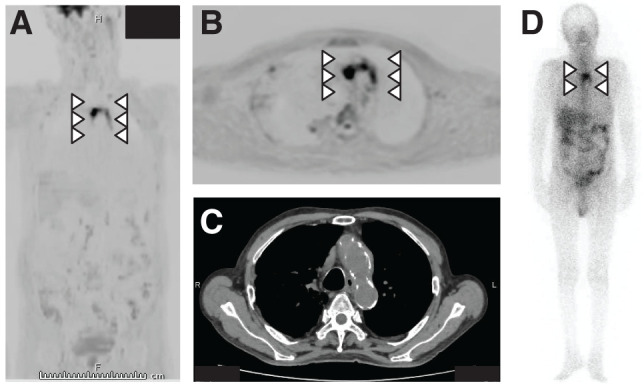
Fig. 2 Diffusion-weighted whole-body imaging with background body signal suppression shows accumulation in the aortic arch (A, B). Computed tomography reveals wall thickening of the aortic arch and slight aneurysmal enlargement (C). Gallium scintigraphy shows accumulation in the aortic arch (D).
